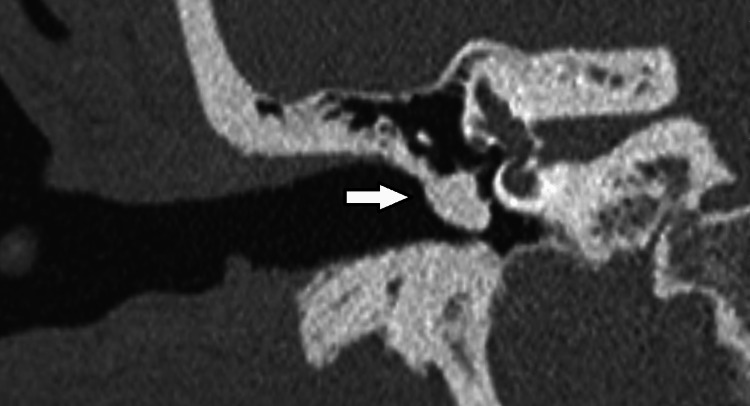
Coronal view of the right ear on CT imaging. Arrow highlights an opacification enveloping handle of the malleus, long process of the incus, and head of the stapes.
